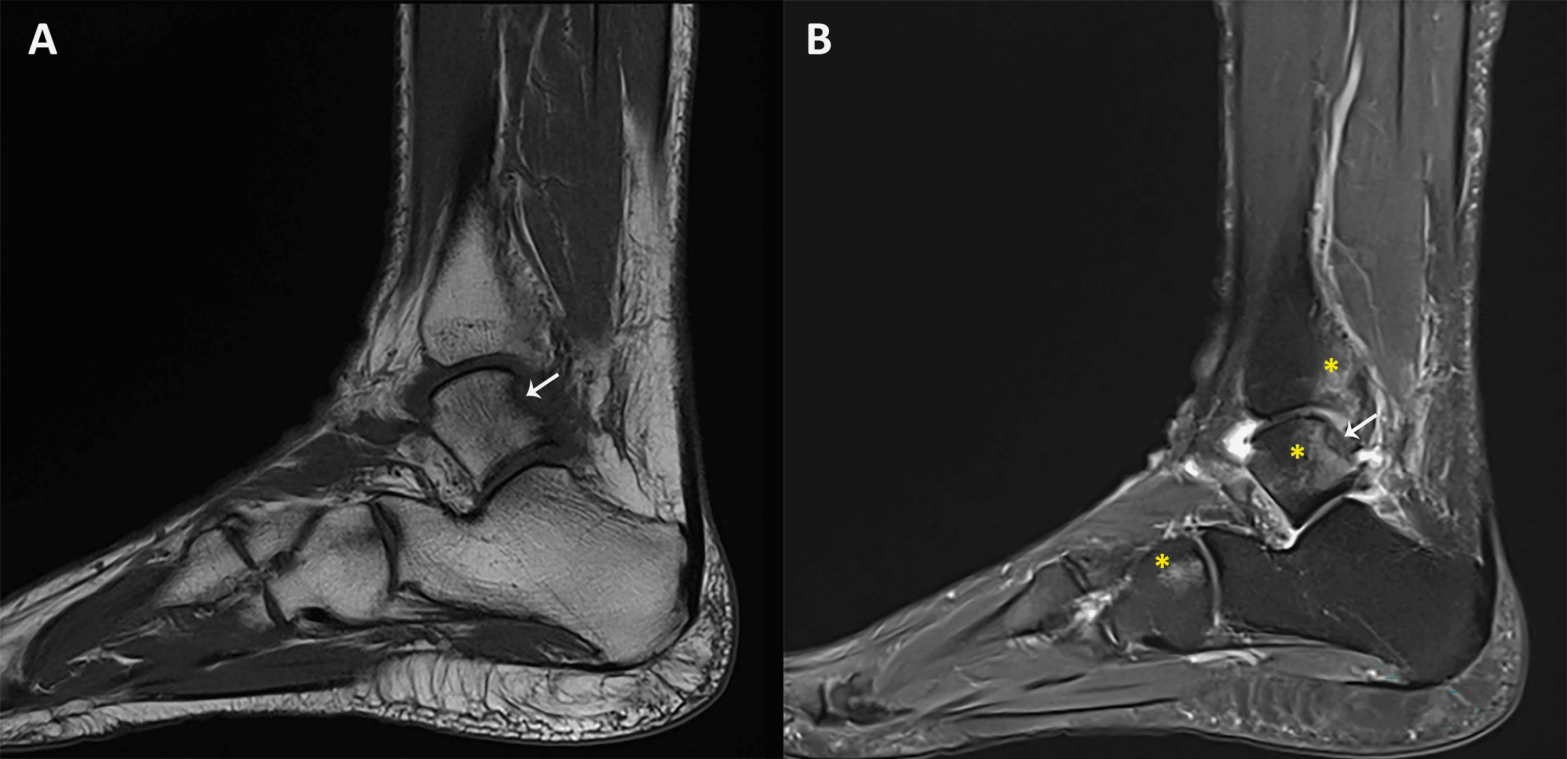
Sagittal MRI views of the ankle demonstrating a subchondral fracture and associated bone contusion. (A) T1-weighted image showing a subchondral fracture of the posterosuperolateral aspect of the talar dome (white arrow). (B) Proton density fat-saturated image highlighting bone contusion edema in the talus, cuboid, and distal tibia (yellow asterisk).
